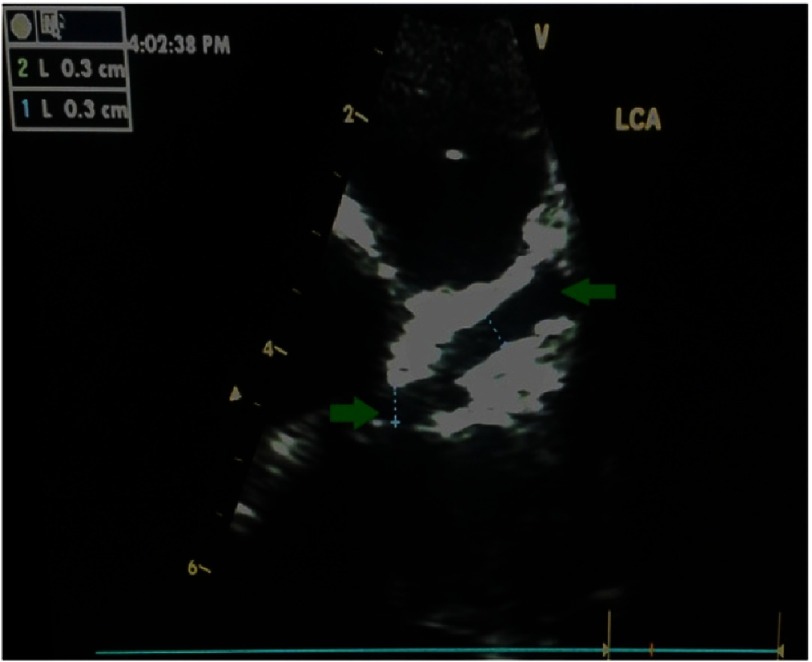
Two-dimensional echocardiography of case 3 showing saccular dilatation of the origin of left main coronary artery (LCA) (arrow) and aneurysmal dilatation of left anterior descending (LAD) artery.
